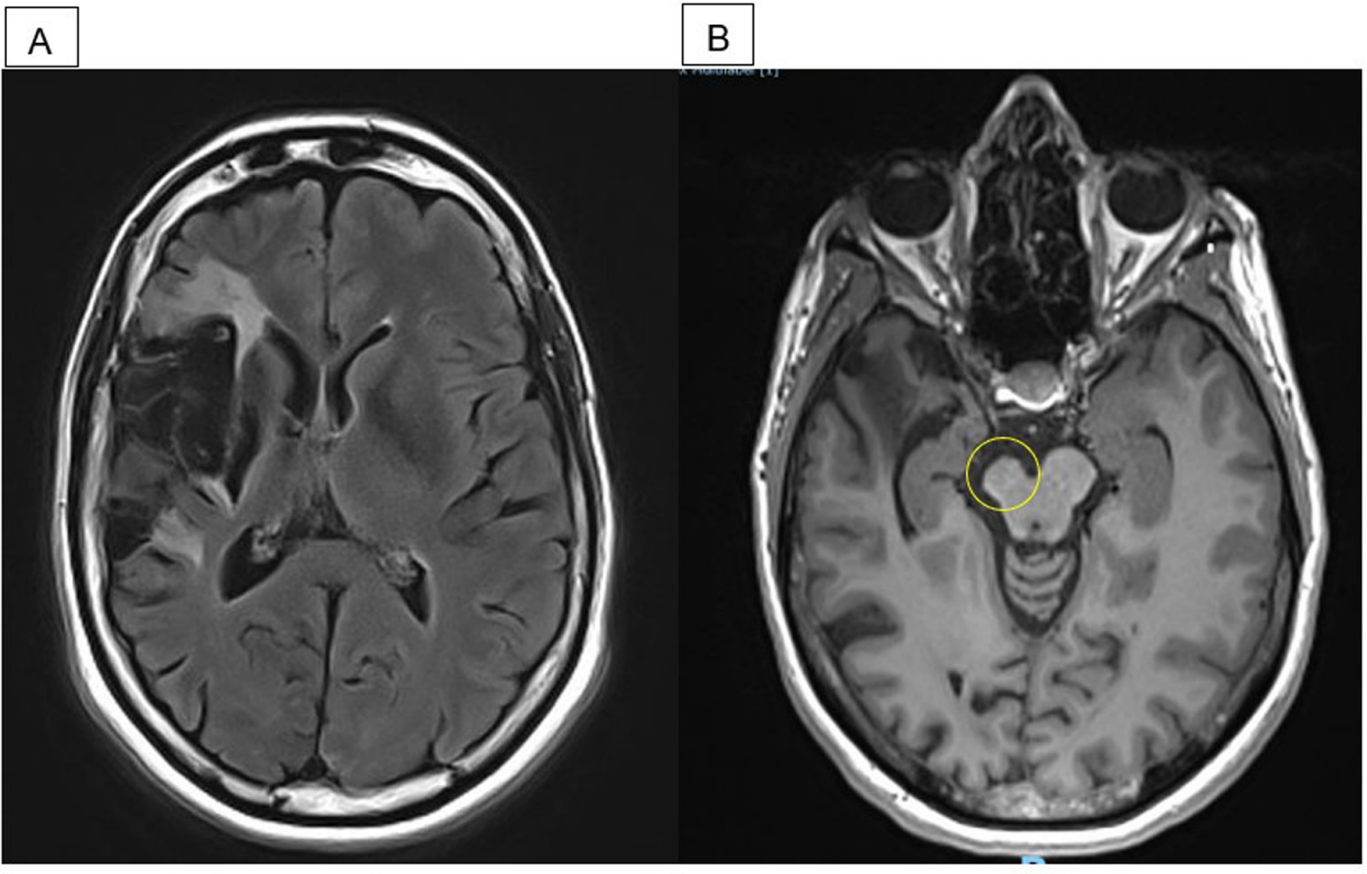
MRI performed in April 2019, 5.5 years poststroke and 4 months after the end of guided self-rehabilitation contract (GSC). (A) Axial flair: right middle cerebral artery infarct. (B) Axial T1: right midbrain atrophy.
